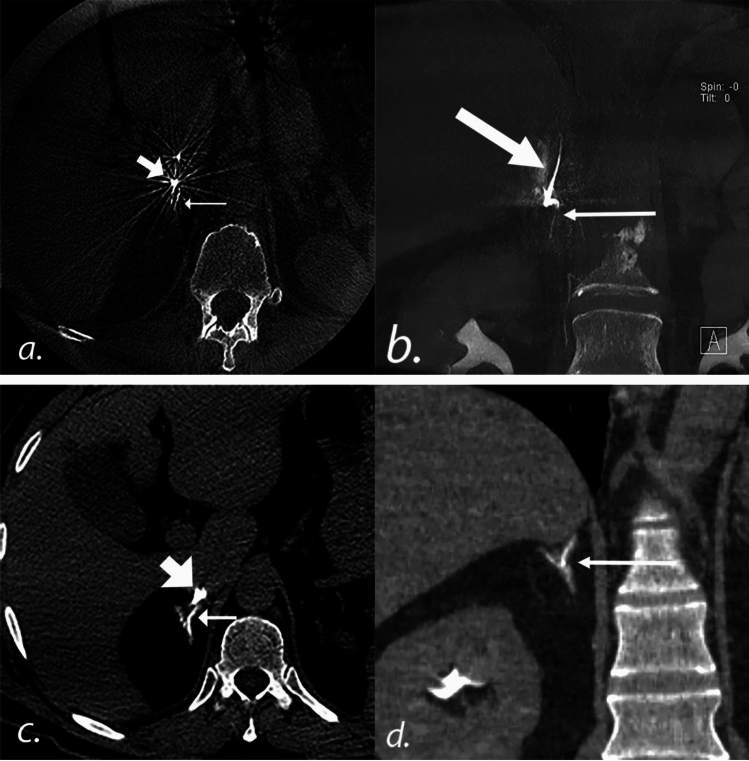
Intraprocedural CT images of the RAV during AVS. a 65-year-old male was referred for AVS. Axial image from an intraprocedural CBCT scan showing the RAV (*thin arrow*). Steak artifact is caused by the radiopaque marker at the tip of the C2 catheter (*thick arrow*) which is used to cannulate the RAV. b Coronal reconstruction demonstrates the C2 catheter (*thick arrow*) and faint contrast within the medial limb of the right adrenal gland (*thin arrow*). c 59-year-old male was referred for AVS. Axial image from MDCT demonstrates the RAV (*thin arrow*). While the tip of the C2 catheter (*thick arrow*) is radiopaque, the degree of streak artifact is less pronounced than on CBCT. d Coronal reconstruction demonstrates contrast clearly opacifying the entirety of the adrenal gland (*thin arrow*). *RAV* right adrenal vein, *AVS* adrenal venous sampling, *CBCT* cone-beam CT, *C2* Cobra-2, *MDCT* multidetector CT
